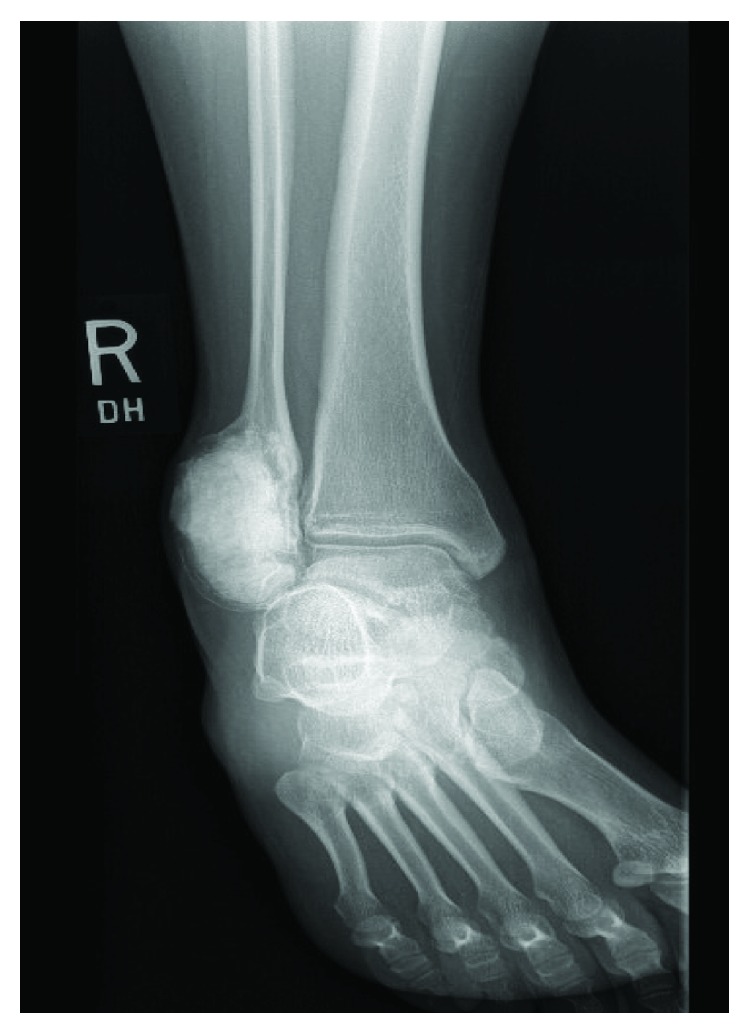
Mortise radiographs taken at 3 years following denosumab treatment and surgery demonstrating no local recurrence.
